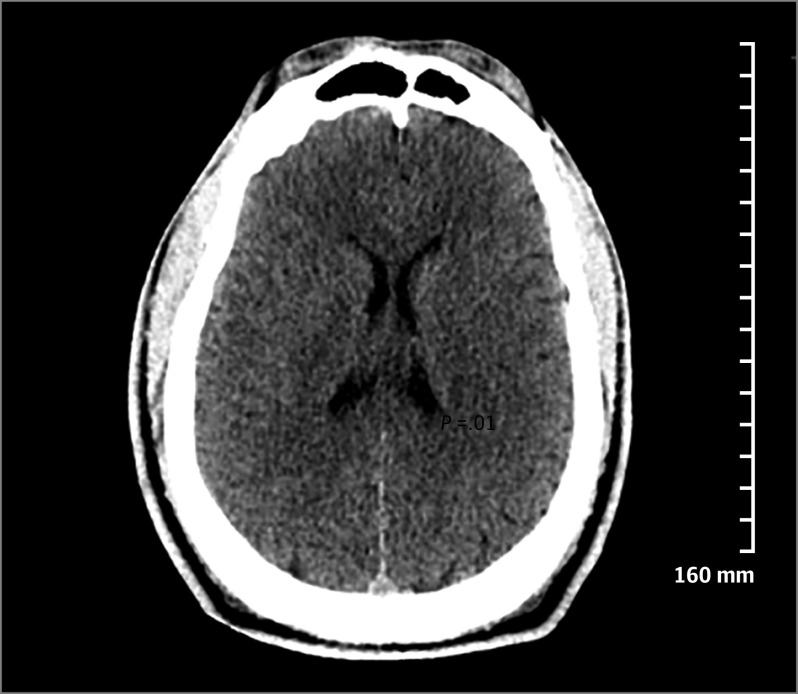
Computed Tomography Image of Patient With Altered Mental Status and Seizure Computed tomography of head was obtained without intravenous contrast agent. Note the diffuse cerebral edema and loss of gray-white differentiation. The patient self-extubated within 3 days after his presentation (patient 16 in Table 2).
